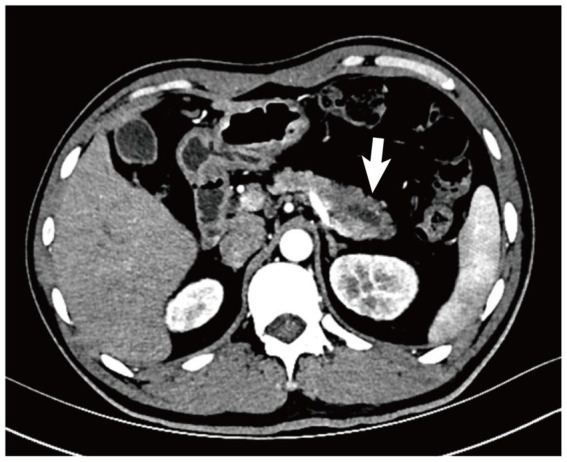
Abdominal enhanced computed tomography (CT) showed thickening of the pancreatic tail with hypodense shadows, suggesting a suspicious pancreatic mass (white arrow head).
